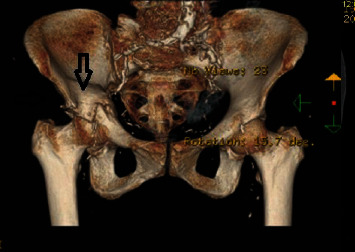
CT reconstruction image showing the transverse fracture line in the right hip. Note the proximity of the right iliac screw to the acetabular roof. Correct position of the iliac screws within the laminae of the pelvic bone bilaterally.
